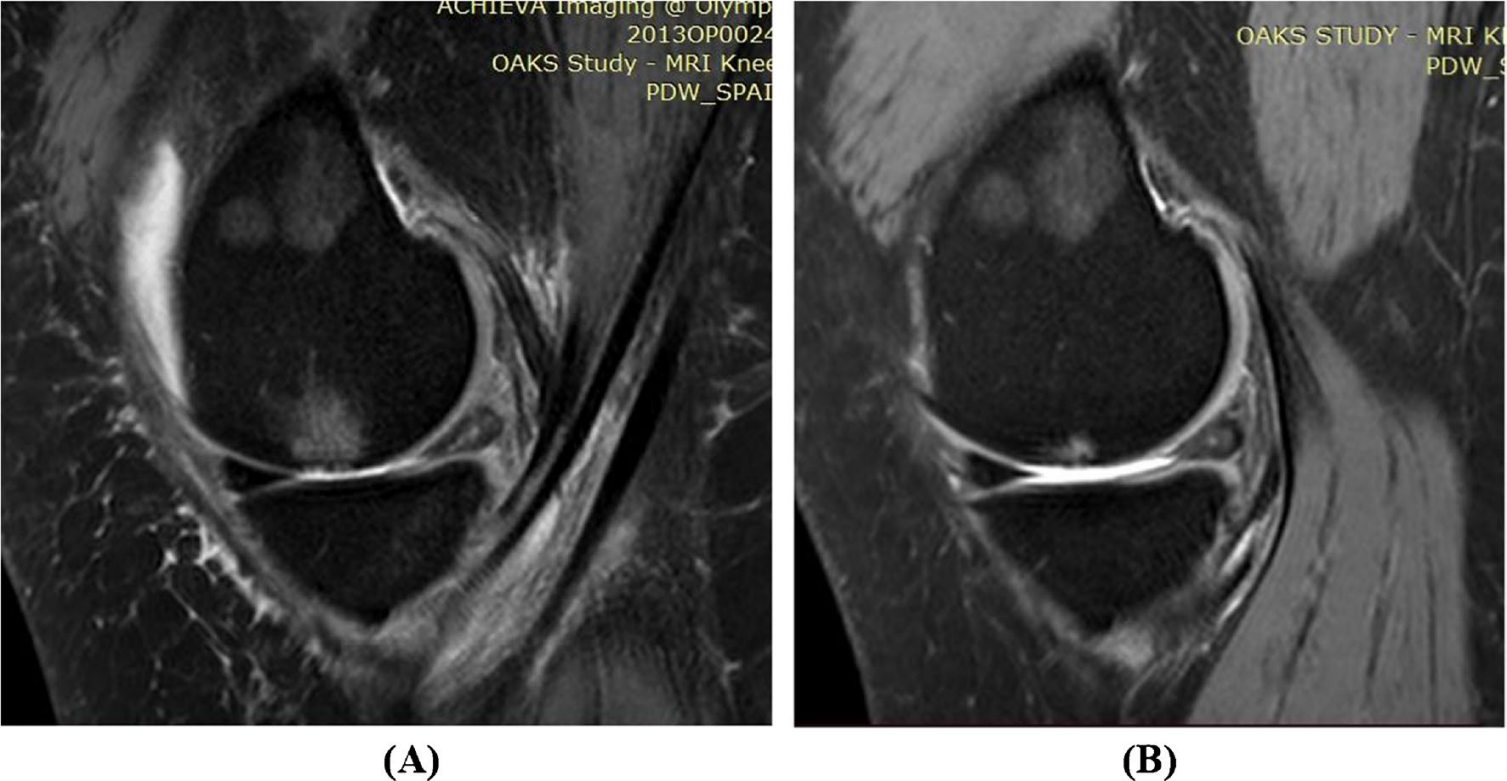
Bone marrow lesions at baseline and follow-up. A Baseline MRI, showing a grade 3 bone marrow lesion in the medial femur; B follow-up MRI of the same participant, showing a grade 1 bone marrow lesion in the same subregion
